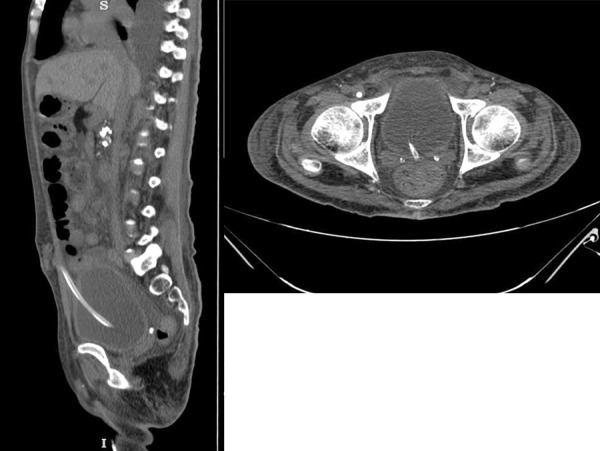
Plain CT abdomen demonstrating the PD catheter perforating the bladder anteriorly and exiting through the posterior surface.
